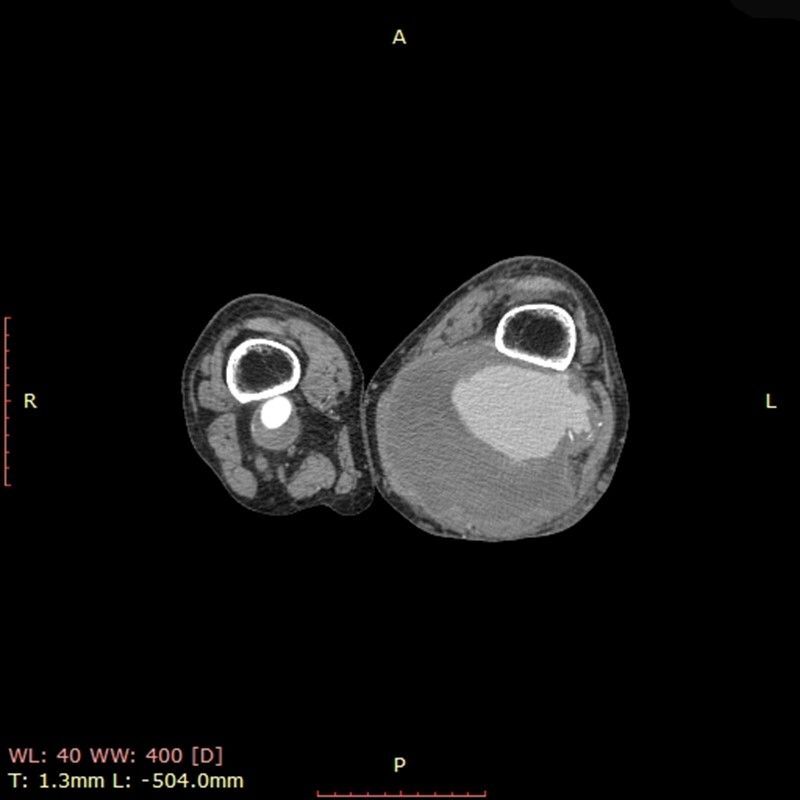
Lower limb computed tomography angiography with contrast showing left and right popliteal artery aneurysms. The left aneurysm has ruptured.
